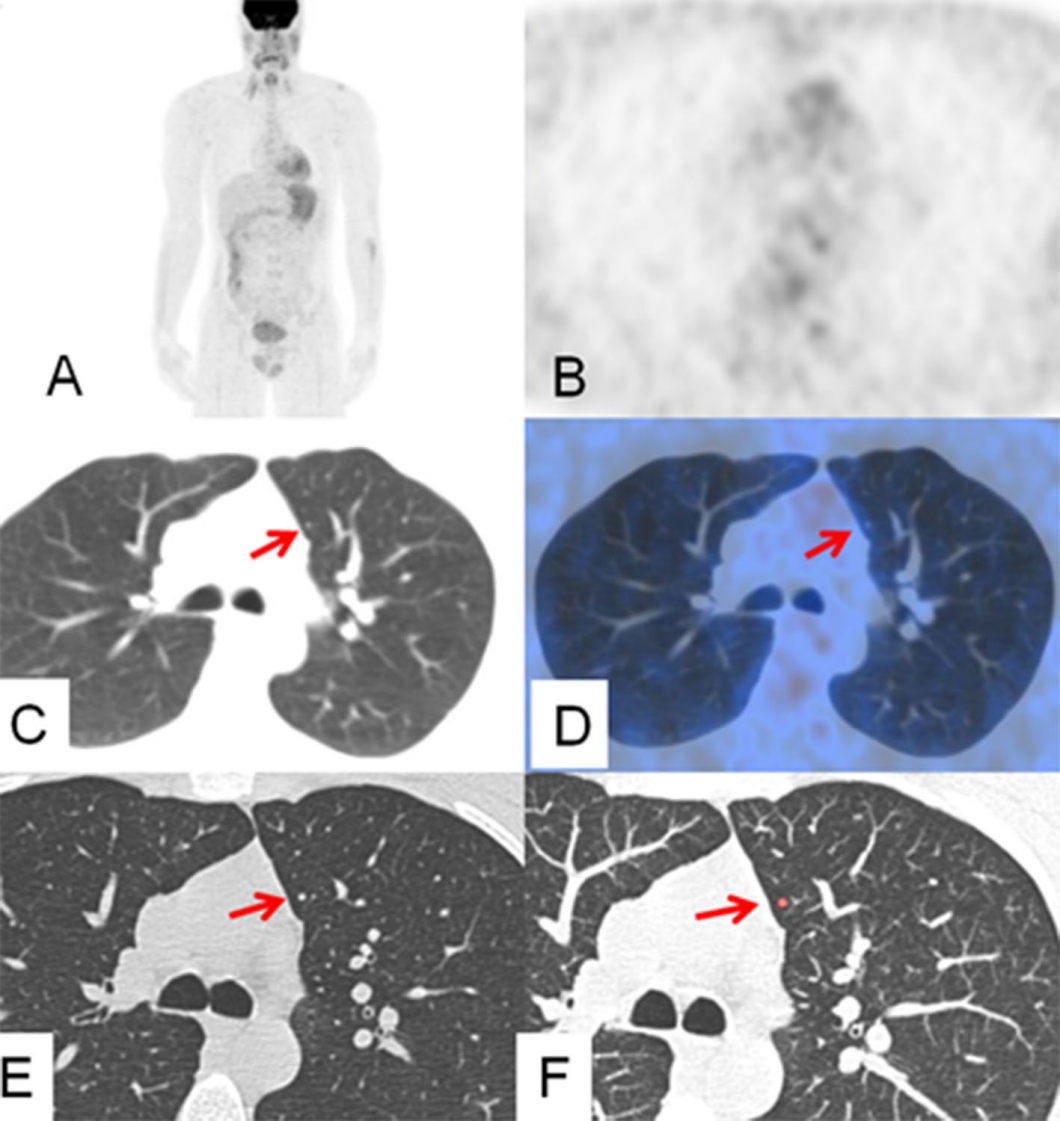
Images of the same patient showing a small nodule in low-dose CT (C) and thin-slice diagnostic CT (E) (arrow) in the upper left lobe without visible uptake in MIP (A), PET (B) and PET/CT (D) images. The nodule was missed by the readers in all CT images and only detected with CAD (F, arrow)
